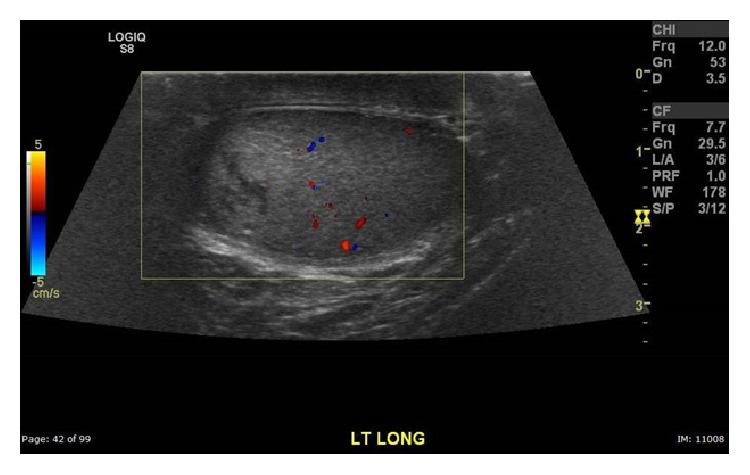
Color-Doppler ultrasound image of the left testis shows an avascular echogenic lesion with hypoechoic center.
